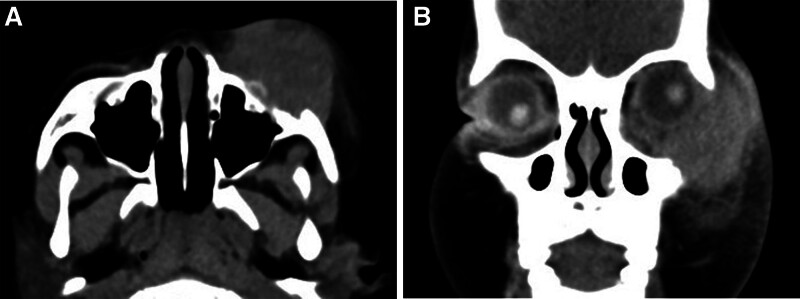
Orbital CT showed a well-defined cystic lesion in the preseptal tissues of the left eyelid, with an incomplete bone structure at the lower margin of the orbit. (A) Axial CT scan; (B) Coronal CT scan. CT = computed tomography.
